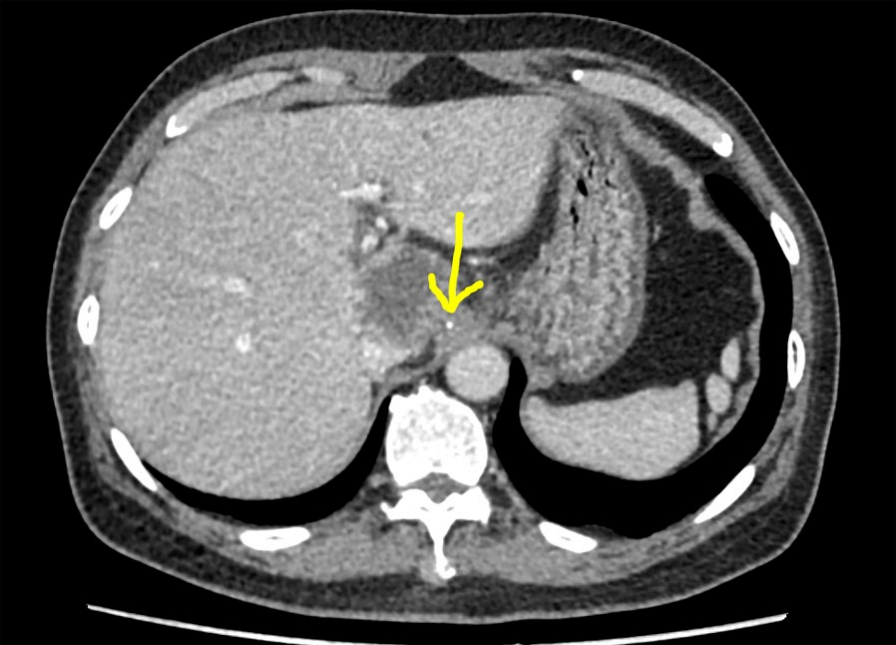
Enhanced computed tomography (CT) revealed an abscess in the caudate lobe of the liver and a needle-like foreign body near the cardia
